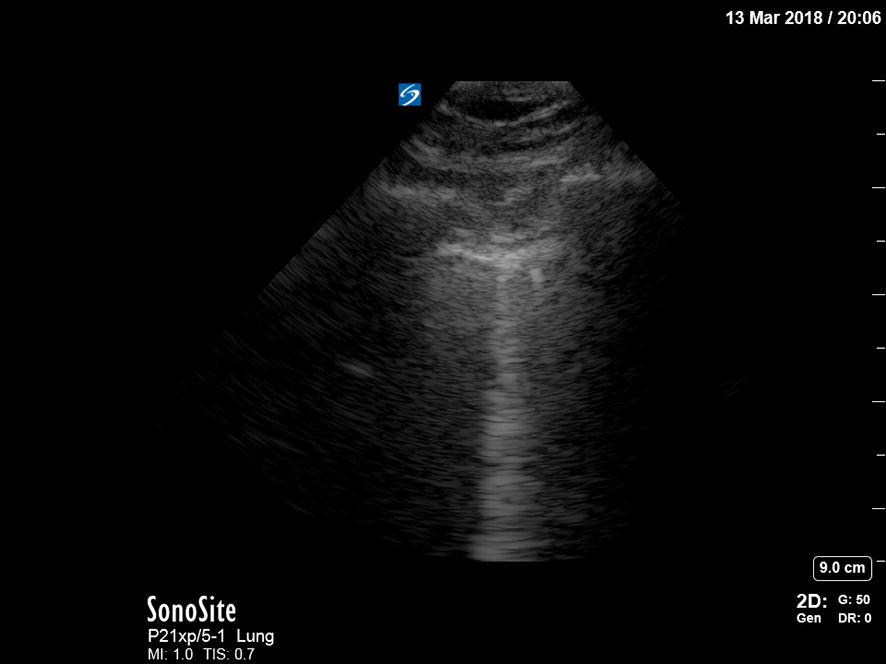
Normal lung + B-line. A normal lung ultrasound image acquired with a 5–1 MHz phased-array cardiac transducer. In the middle of the image, one B-line is seen. This is also seen in healthy subjects and a single B-line is without meaning
